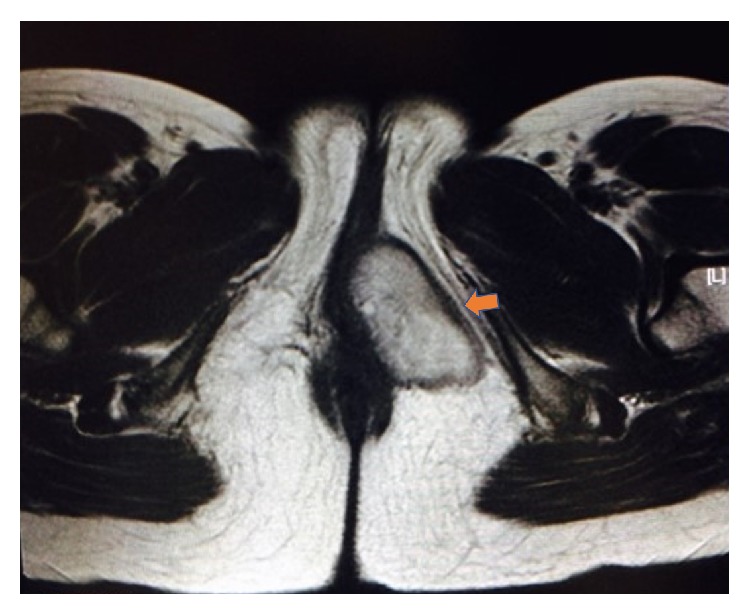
Magnetic resonance imaging of the pelvis showed a well-circumscribed mass in the vulvar subcutaneous region.
